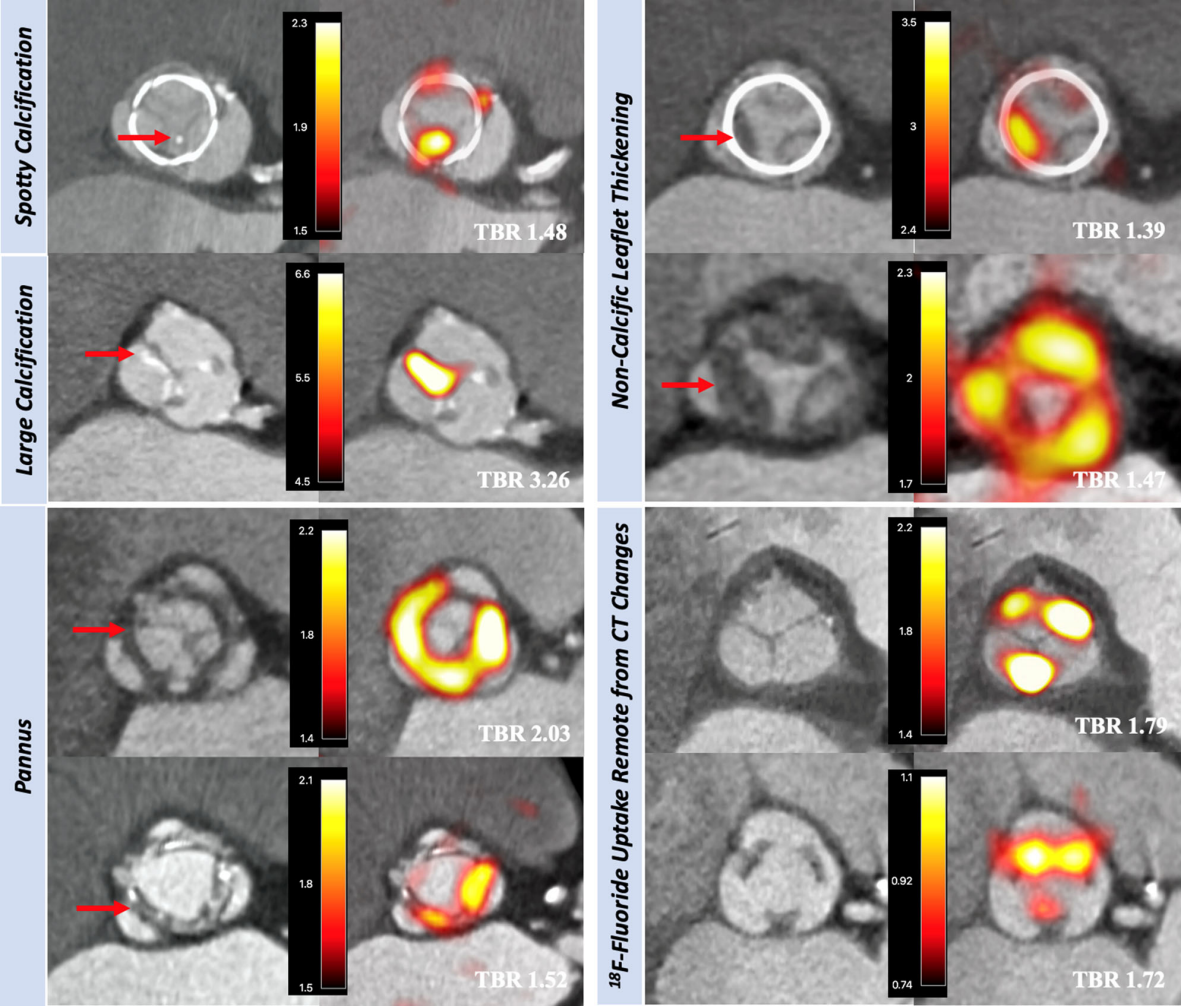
Baseline CT (left) and ^18^F-fluoride PET (right) images from patients with bioprosthetic aortic valves. En face CT images of aortic bioprosthetic valves showing spotty calcification and large calcification (top left), circumferential pannus (bottom left), and noncalcific leaflet thickening suggestive of thrombus (top right) (all abnormalities identified by red arrows). Hybrid en face PET-CT images in the same patients: increased bioprosthetic ^18^F-fluoride activity (red/yellow areas) is observed in each patient colocalizing with the CT abnormalities. ^18^F-fluoride activity was also commonly observed remote from leaflet changes on CT (bottom right). Scale bars in the center of each pair of images represent standardized uptake values (SUV). Target-to-background (TBR) values are annotated on the hybrid PET-CT images in white text. Adapted from Timothy et al.^38^ Under creative commons licence
